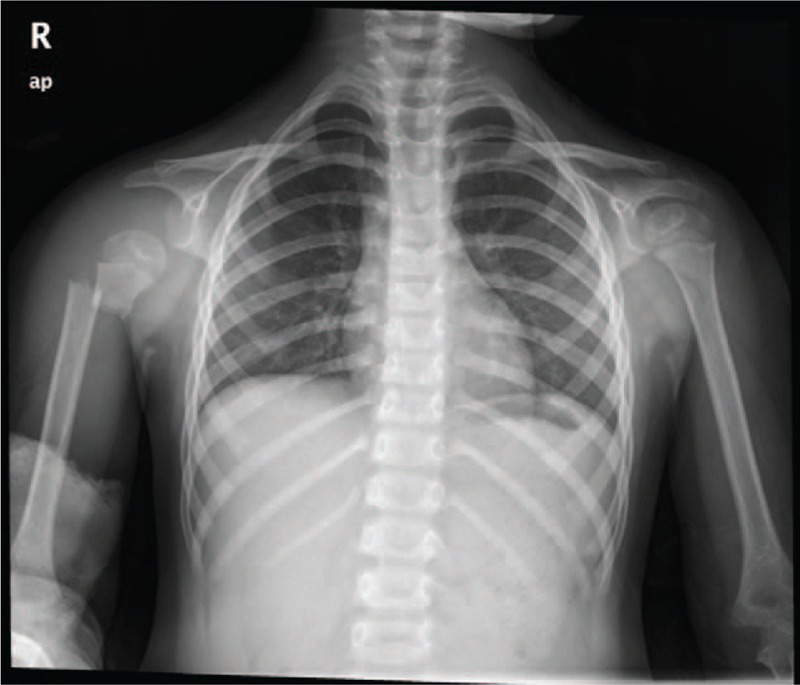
Preoperative bilateral anteroposterior shoulders x-ray showing a combination of right proximal humerus fracture with shoulder dislocation.
